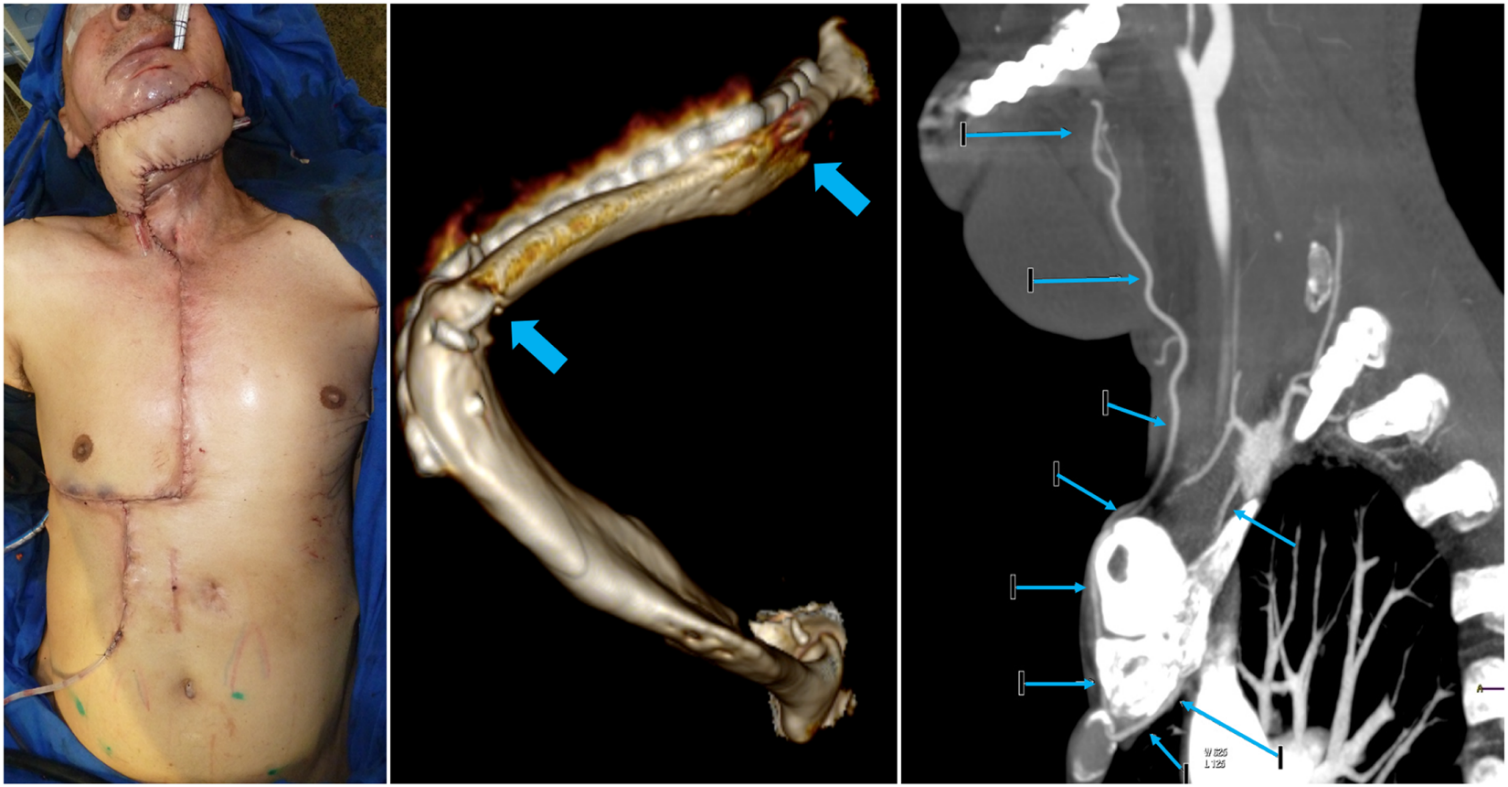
Post-op clinical cases. (A) Primary closure of the PIMOC donor site in patient 3. (B) 3D reconstruction of computed topography angiography (CTA) demonstrating osseous integration of the left mandibular body with the 7^th^ rib (blue arrows) in patient 4. There is increased ossification at 6 months post-op. (C) Sagital CTA demonstrating the internal mammary artery pedicle pivoted anteriorly around the first rib (A) and clavicle (B) and ascending into the head and neck region (blue arrows).
